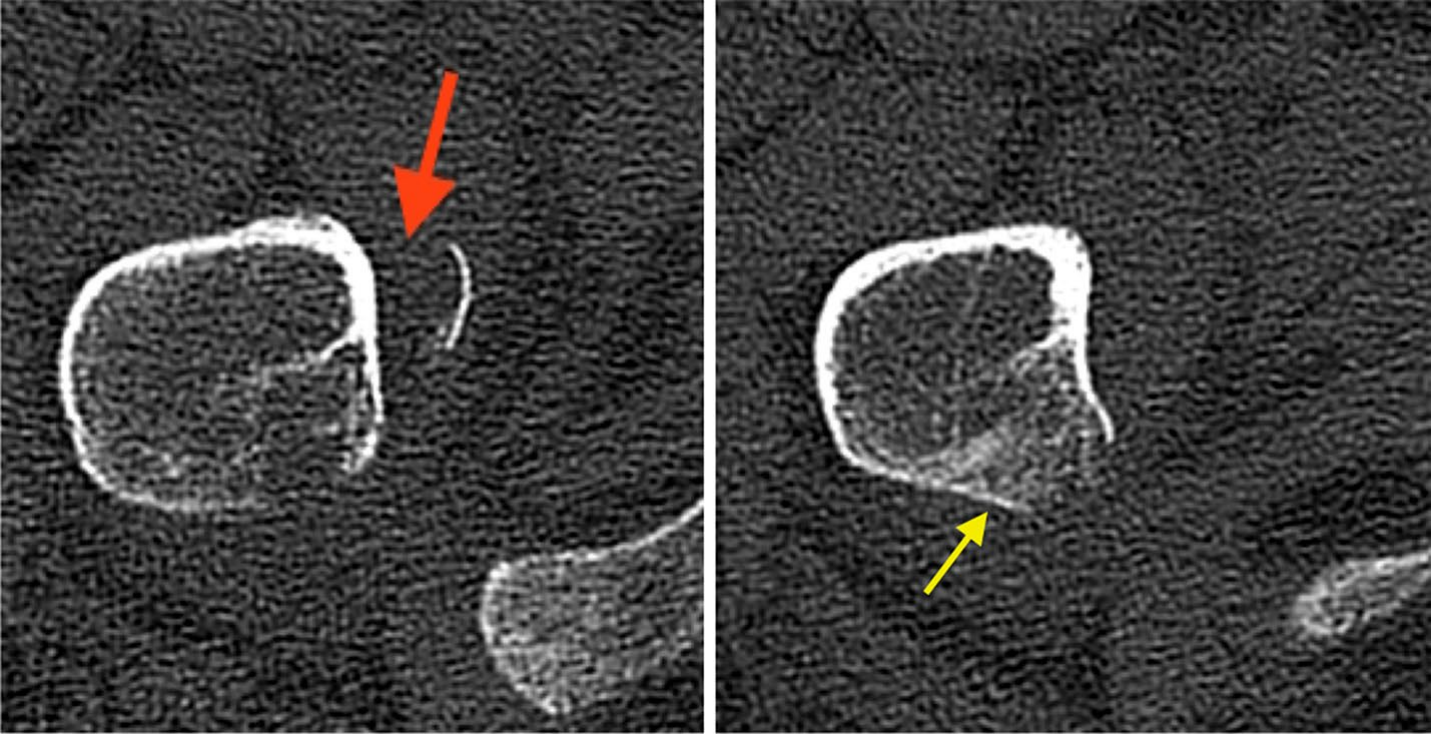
CT scan of the right trochanter area and reconstruction in axial view. Avulsion fracture of the lesser trochanter (*red arrow*) and majority of the lesser trochanter is intact (*yellow arrow*)
